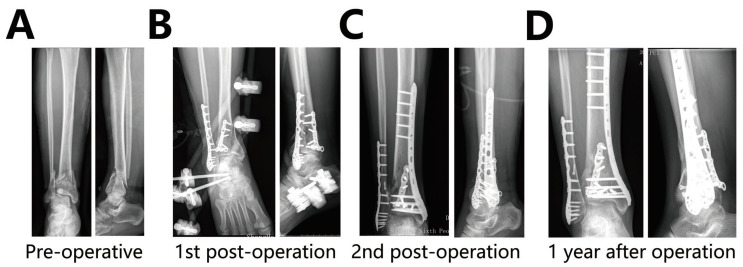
(A) Pre-operative X-ray. (B) The x-rays show the fixation of the posterior tibial column and fibula by a locking plate in the first surgery, combined with external fixator. (C) The x-ray shows the fixation of the tibia with a medial locking plate during the second surgery. (D) The x-ray shows the healing of the patient’s fracture one year after surgery.
